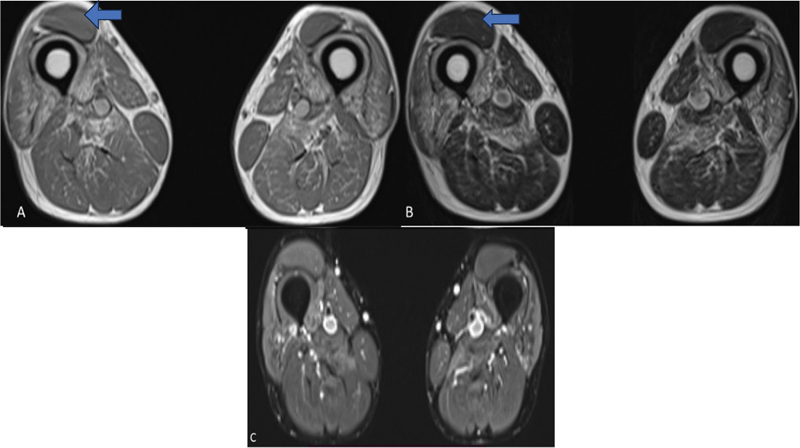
Muscle MRI of the patient. (
